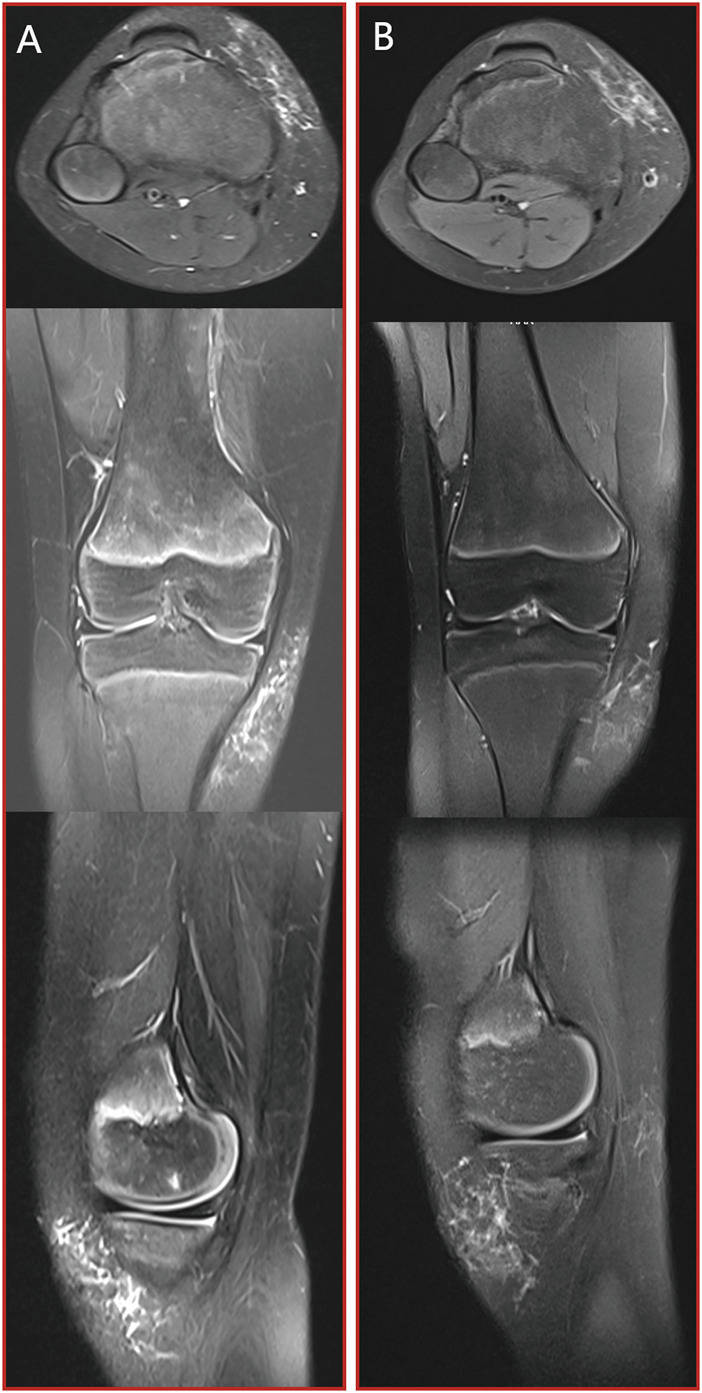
Case 1: patient with microcystic LMs of the right knee which achieved a substantial and noteworthy improvement after a single session of combination therapy. (A) pre-treatment MRI stud; (B) post-treatment MRI study. From top to bottom: axial T2 fat saturated image, coronal T2 fat saturated images and sagittal T2 fat saturated images.
